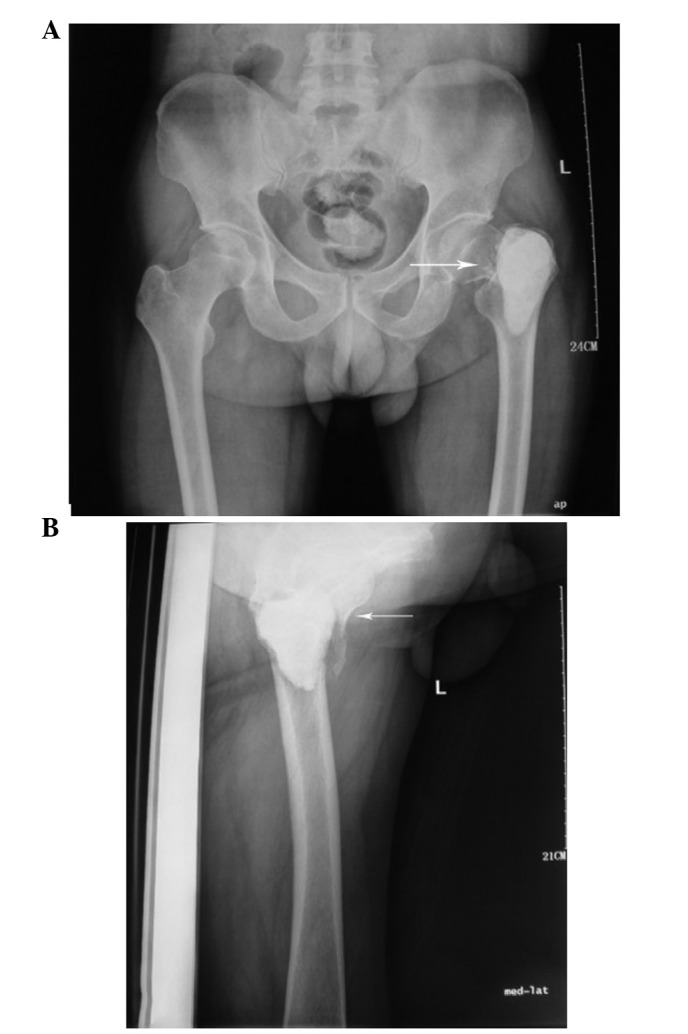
(A) Frontal and (B) lateral x-ray images of the pelvis showing a fracture of the left femoral neck (white arrow).
